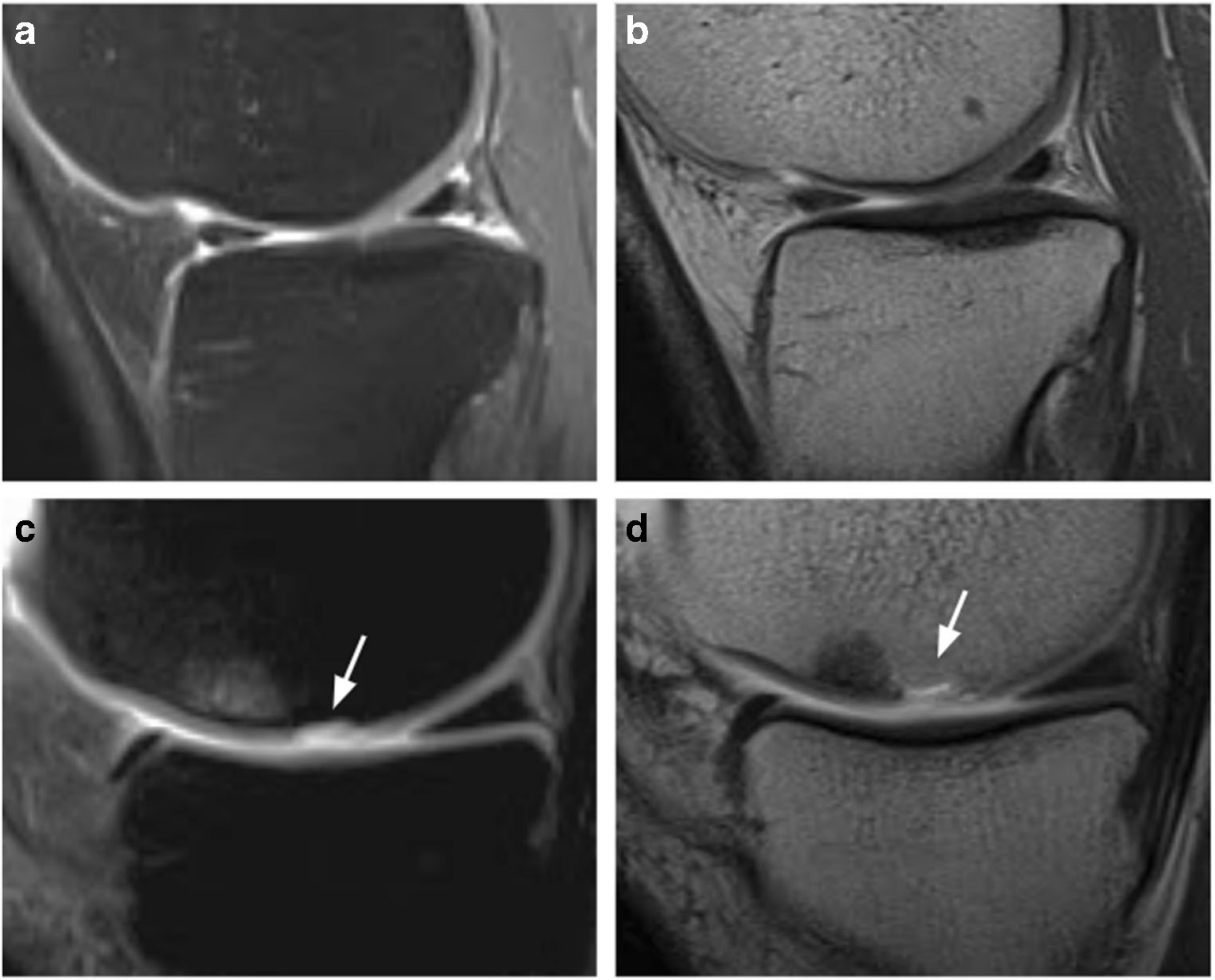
a, b A 39-year-old man treated with MACI of the internal tibial plate at mid-term with mMOCART score of 70 and Cincinnati score of 9. a Sagittal oblique FSE PD fat-saturated image shows non-structural incongruence of the chondral surface. b Sagittal oblique SE T1-weighted image. No contrast enhancement. c, d A 29-year-old woman treated with MACI of the internal femoral condyle at mid-term with mMOCART score of 25, Cincinnati score 9. c Sagittal oblique FSE PD fat-saturated image shows structural incongruence of the chondral surface (arrow) and subchondral bone oedema. d Sagittal oblique SE T1-weighted image. Contrast enhancement in the subchondral bone (arrow) [images obtained with “Unit1”]
